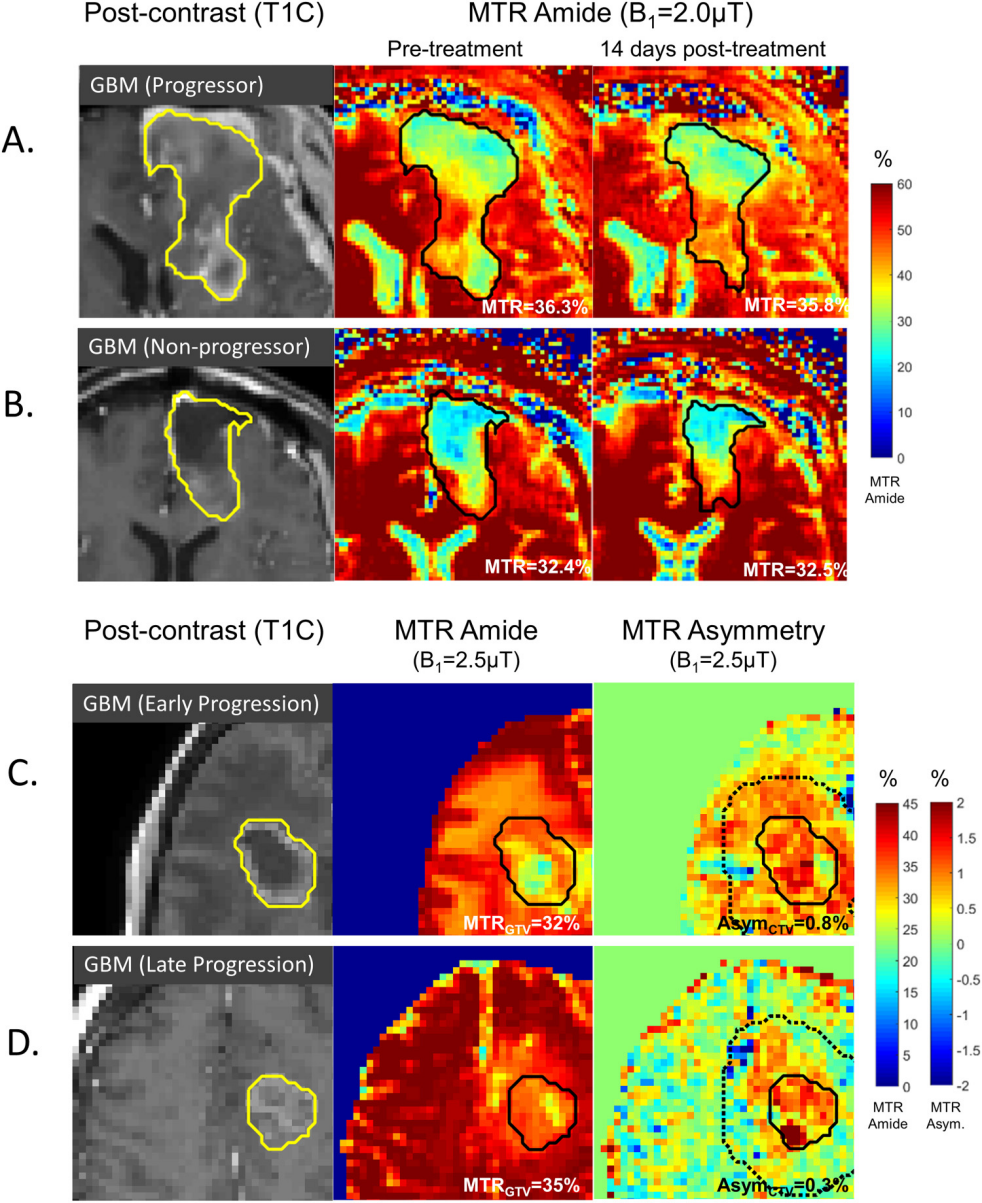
CEST for predicting treatment response in glioblastoma: (A, B) MTR amide maps from single-slice CEST at 3T are shown before treatment at the time of radiation planning MRI (middle column) and at 14 days after the start of radiation (right column) for patients demonstrating early progression in (A) versus late progression in (B). (C, D) Images at the time of radiation planning MRI are shown of the MTR amide map (middle column) and the MTR asymmetry map (right column). The solid lines include the enhancing tumor plus the resection cavity. The dotted lines on the MTR asymmetry maps represent the clinical target volume. Regions of high MTR asymmetry in the CTV were associated with early progression (C). An example of late progression (D) is also shown.
